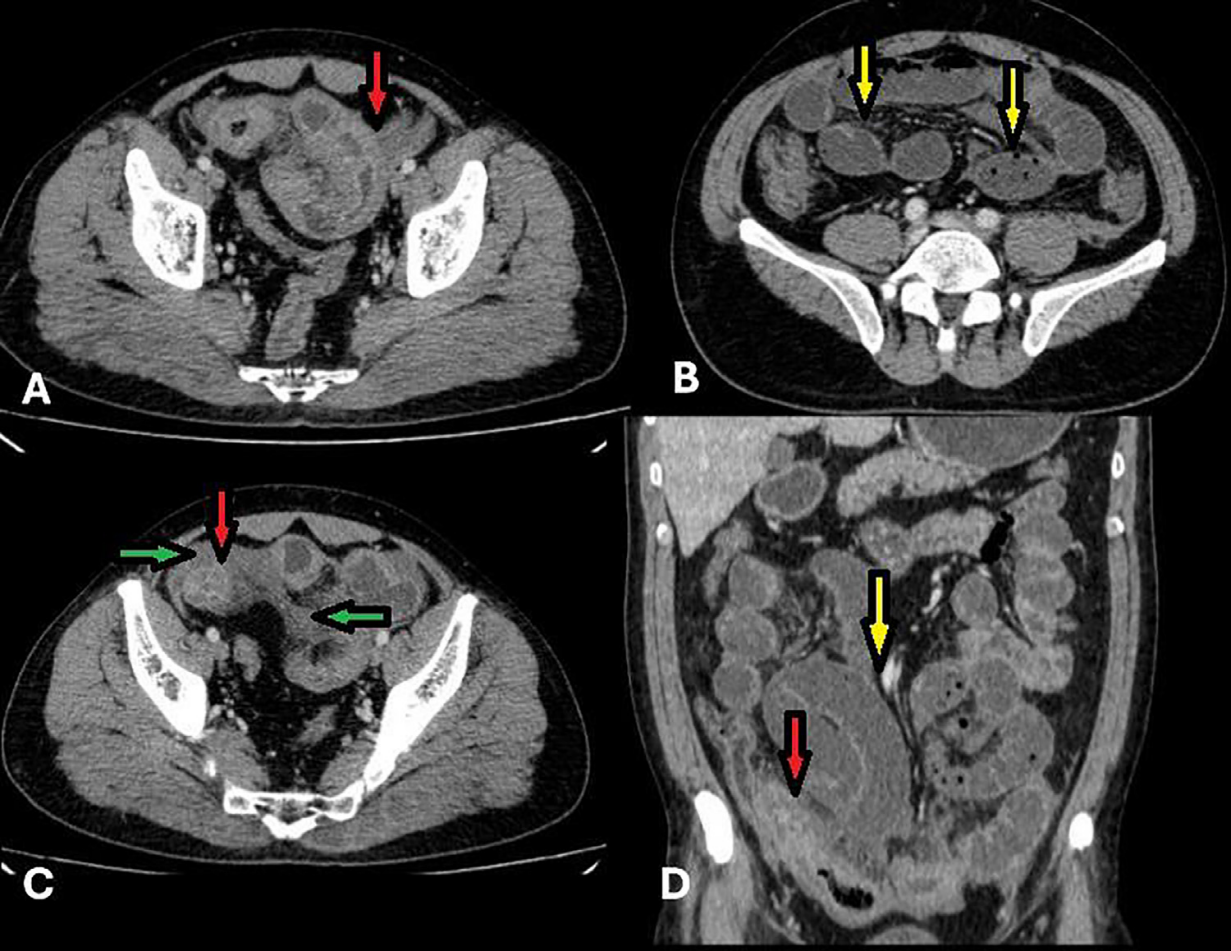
Abdominopelvic computed tomography (CT) with intravenous contrast injection (portal phase). (A) Circumferential long-segment symmetrical wall thickening is observed in the distal ileal loops, appearing hyperdense, suggestive of intramural hematoma (IMH) (red arrows). (B) More Proximal small bowel loops appear dilated, indicating partial obstruction (yellow arrows). Additionally, fluid is seen in mesentery. (C) Hyperdense free fluid is present in the para-colic gutters bilaterally, indicative of mild hemoperitoneum (green arrows). (D) Coronal view confirms circumferential long-segment thickening in the distal ileal loops (red arrows).
